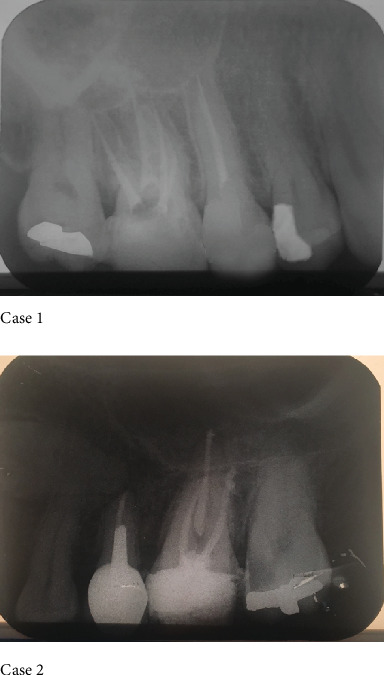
Preapical radiographic of #16 and #26 teeth after root canal therapy.
